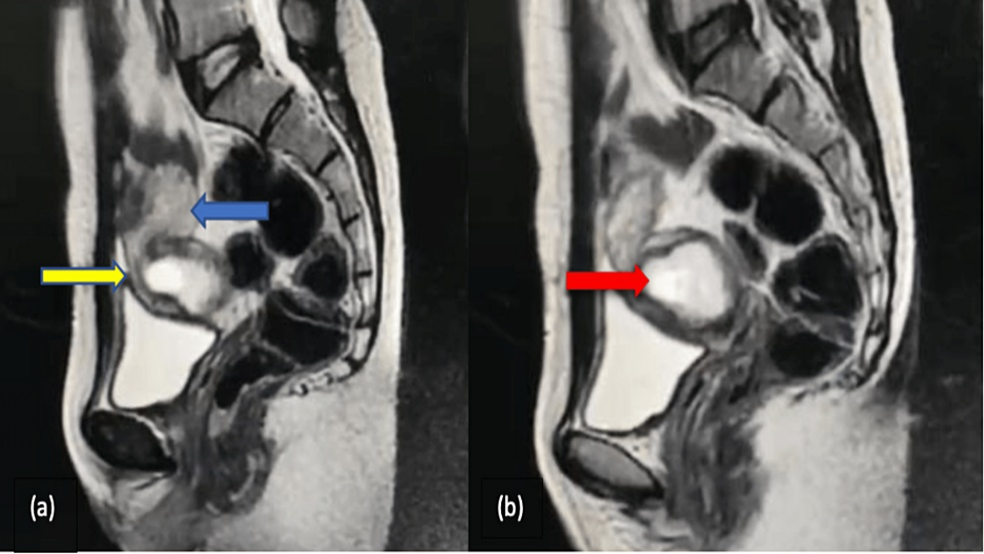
Sagittal T2-weighted MRI showing the (a) uterus (blue arrow) and cervix (yellow arrow), and (b) an irregular gestational sac (red arrow) measuring 4.0 × 2.8 × 3.4 cm is noted in the endocervical canal distal to the internal os appearing hyperintense with an incomplete circumferential hypointense rim. MRI: magnetic resonance imaging
